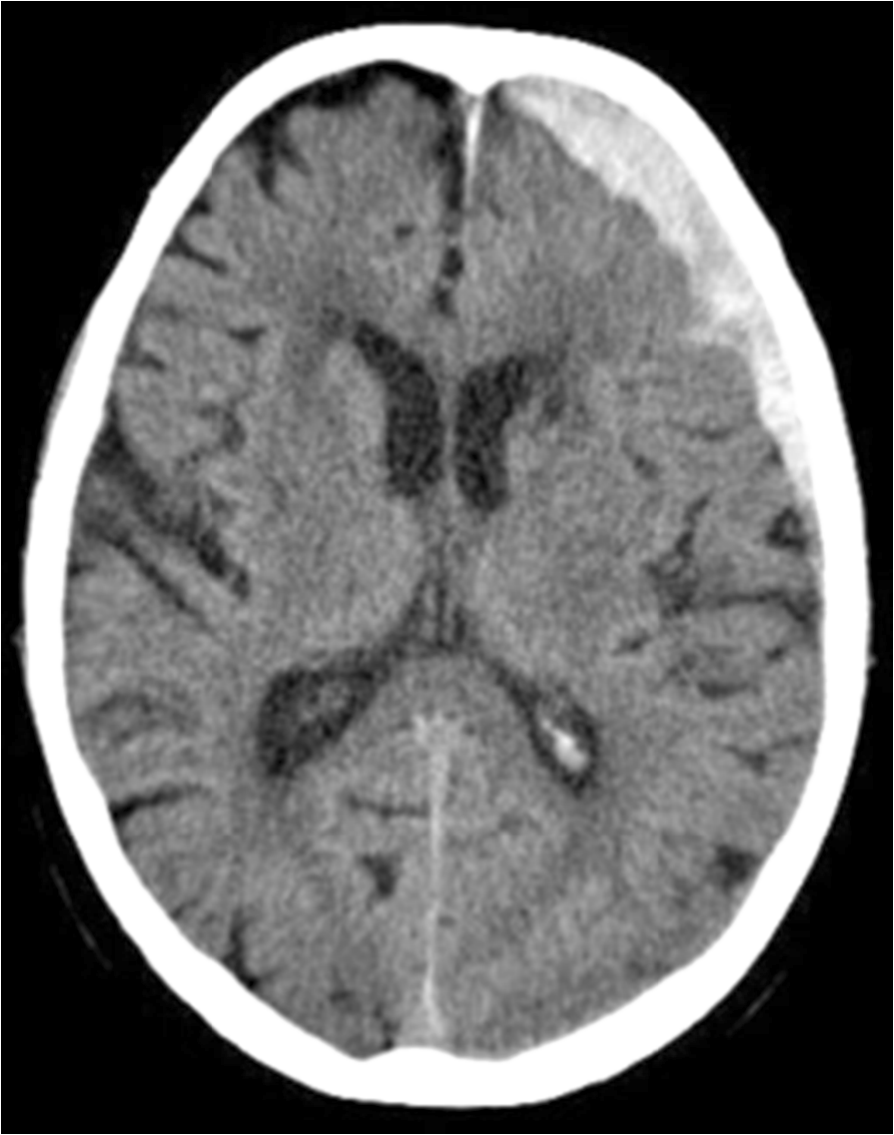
CT scan demonstrating the fronto-temporal subdural haematoma over the left hemisphere.
